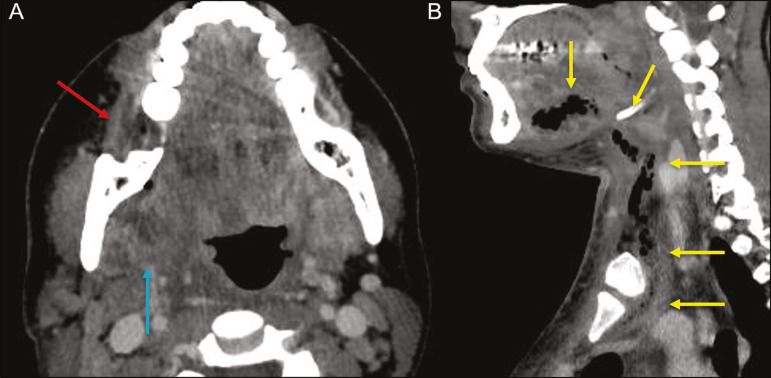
Axial and sagittal CT scans of the face and neck (A and B, respectively) of a patient submitted to extraction of lower right third molar who evolved to the formation of an odontogenic abscess (red arrow). The fluid collection extended to the masticatory space (blue arrow) and, subsequently, to the remaining deep cervical spaces (yellow arrows), to the level of the mediastinum, causing mediastinitis. These findings are compatible with Ludwig's angina.
